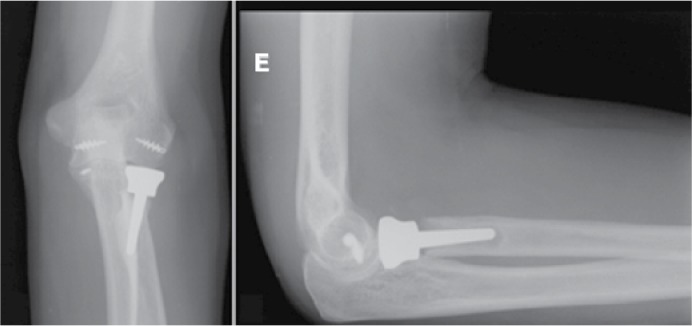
Postoperative incidence X-Ray.
